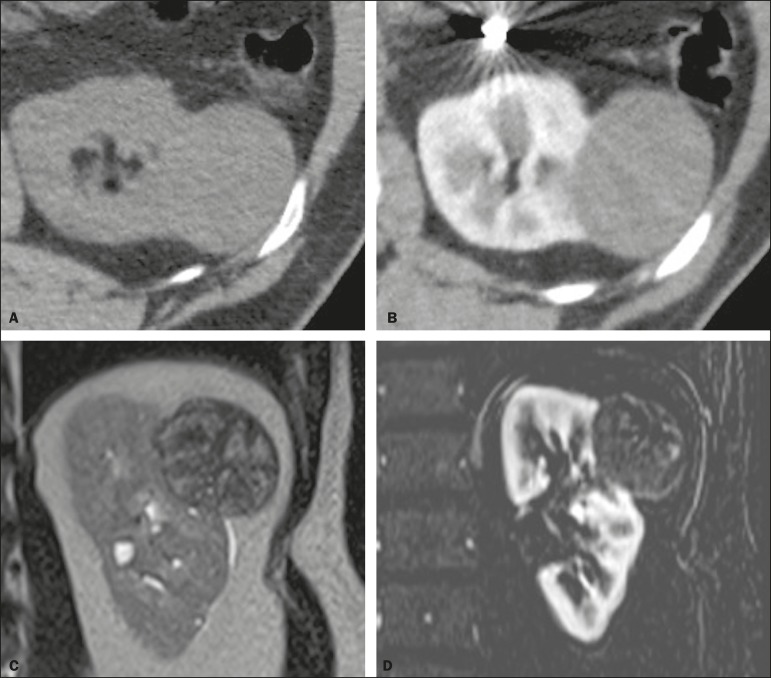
Cystic versus hypovascular mass. A,B: CT showing a hypoattenuating
